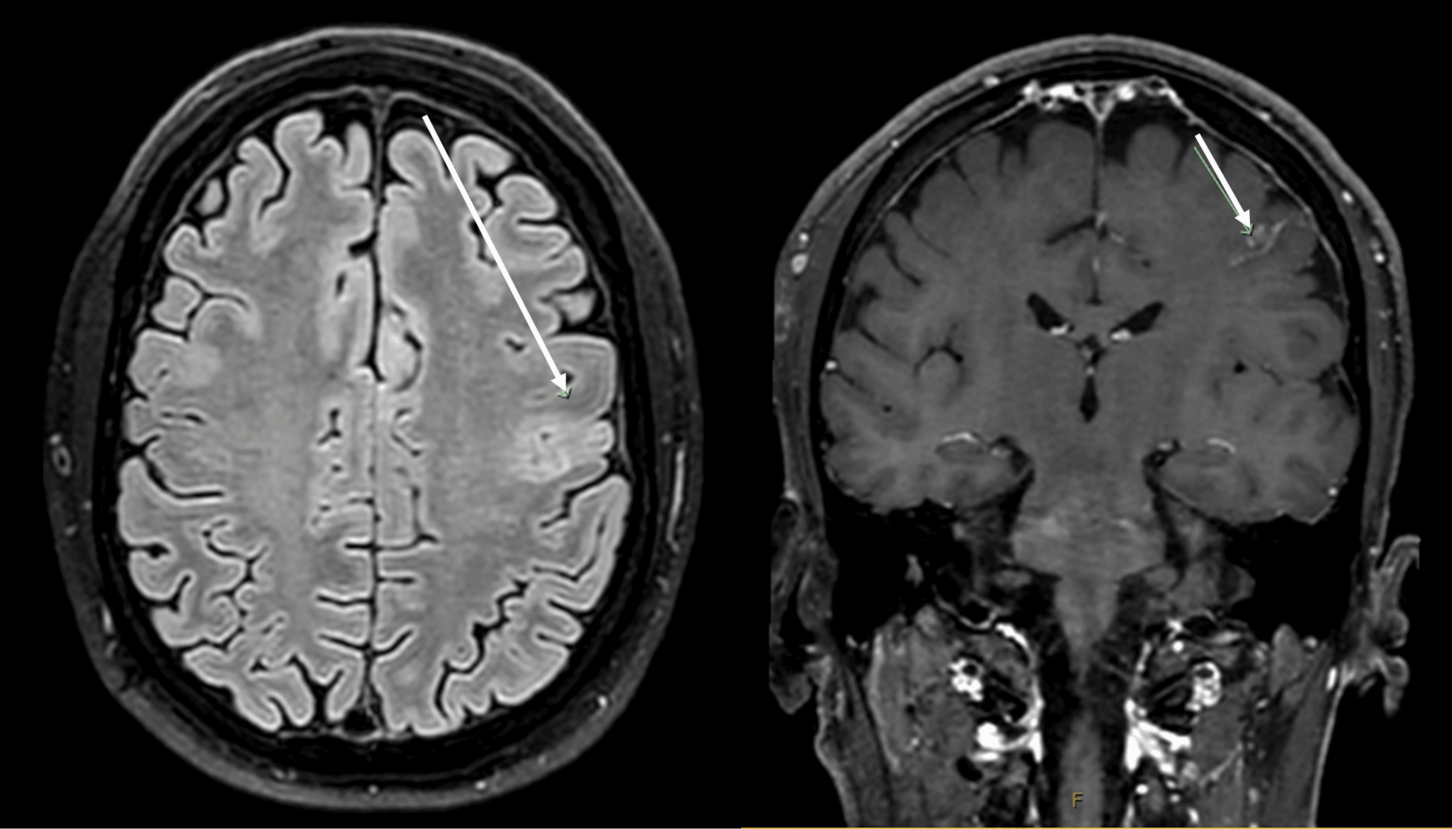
Initial MRI of the brain (16/09/24) showed the focal area of altered F2/fluid-attenuated inversion recovery (FLAIR) signal intensity on the left. The image on the right is a post-contrast T1-weighted (T1W) sequence.
